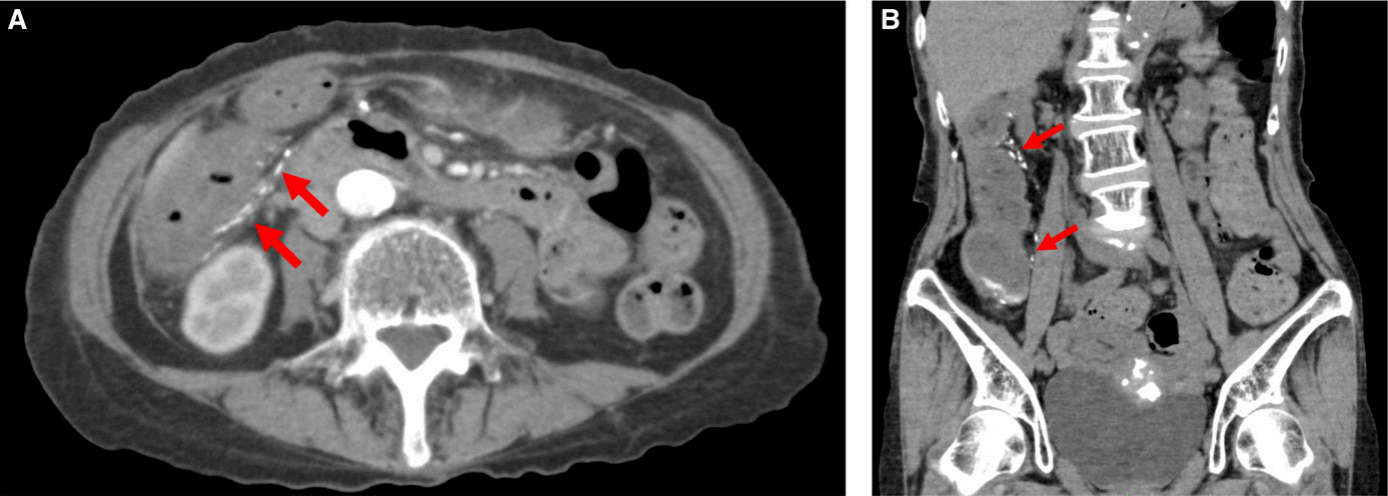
(A) Axial view and (B) coronal view of the abdominal computed tomography scan showing colonic wall thickening with calcification of the superior mesenteric vein in the ascending colon (red arrows in A and B).
